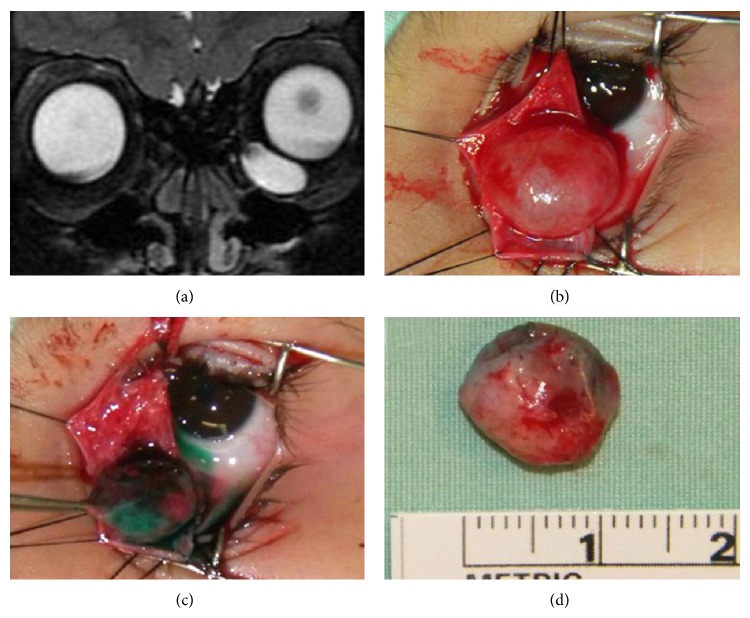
(a) T2 Coronal MRI scan showing a 1.5 cm inferomedial cystic mass in the left orbit. (b) Cyst exposure through a conjunctival incision. (c) Capsule demonstration after intralesional ICG injection. (d) The completely excised cyst.
